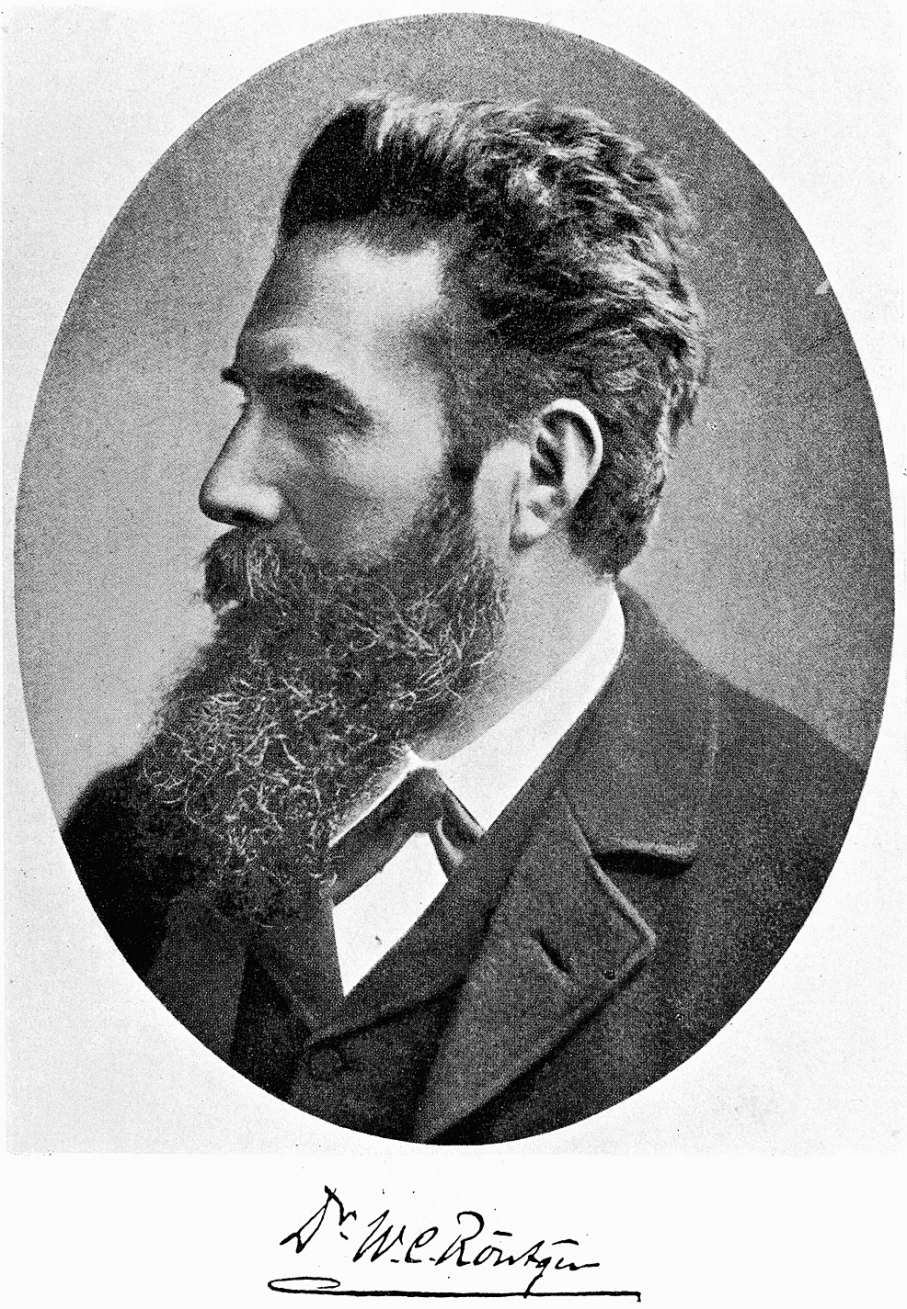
Wilhelm Conrad Röntgen Permission has been granted to use the image. Credit: Portrait of W. C. Roentgen. Wellcome Collection. Attribution 4.0 International (CC BY 4.0).
